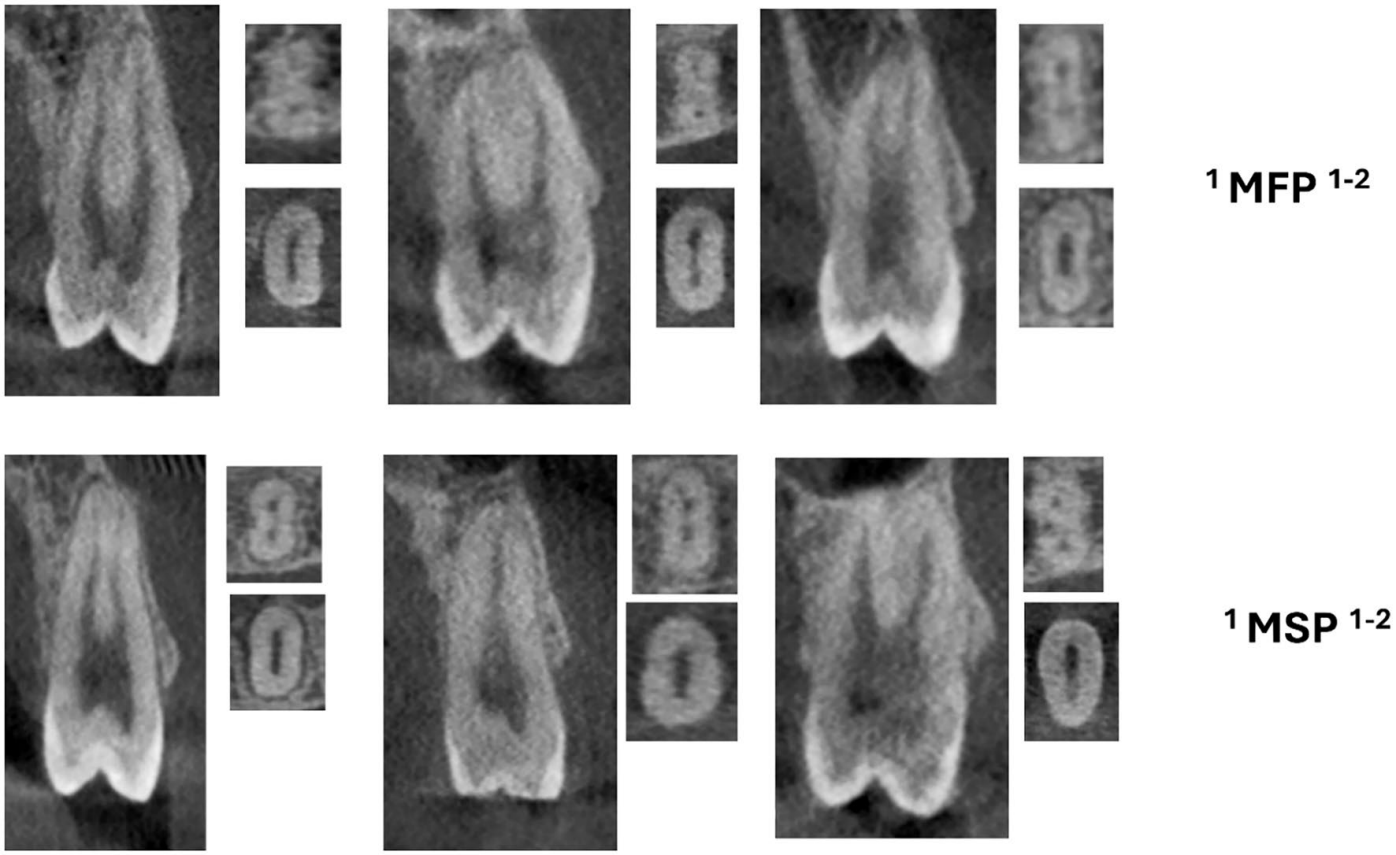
CBCT images (sagittal and axial views) of maxillary premolar (MFP—first premolars, MSP—second premolars) showing two code root canal morphology
